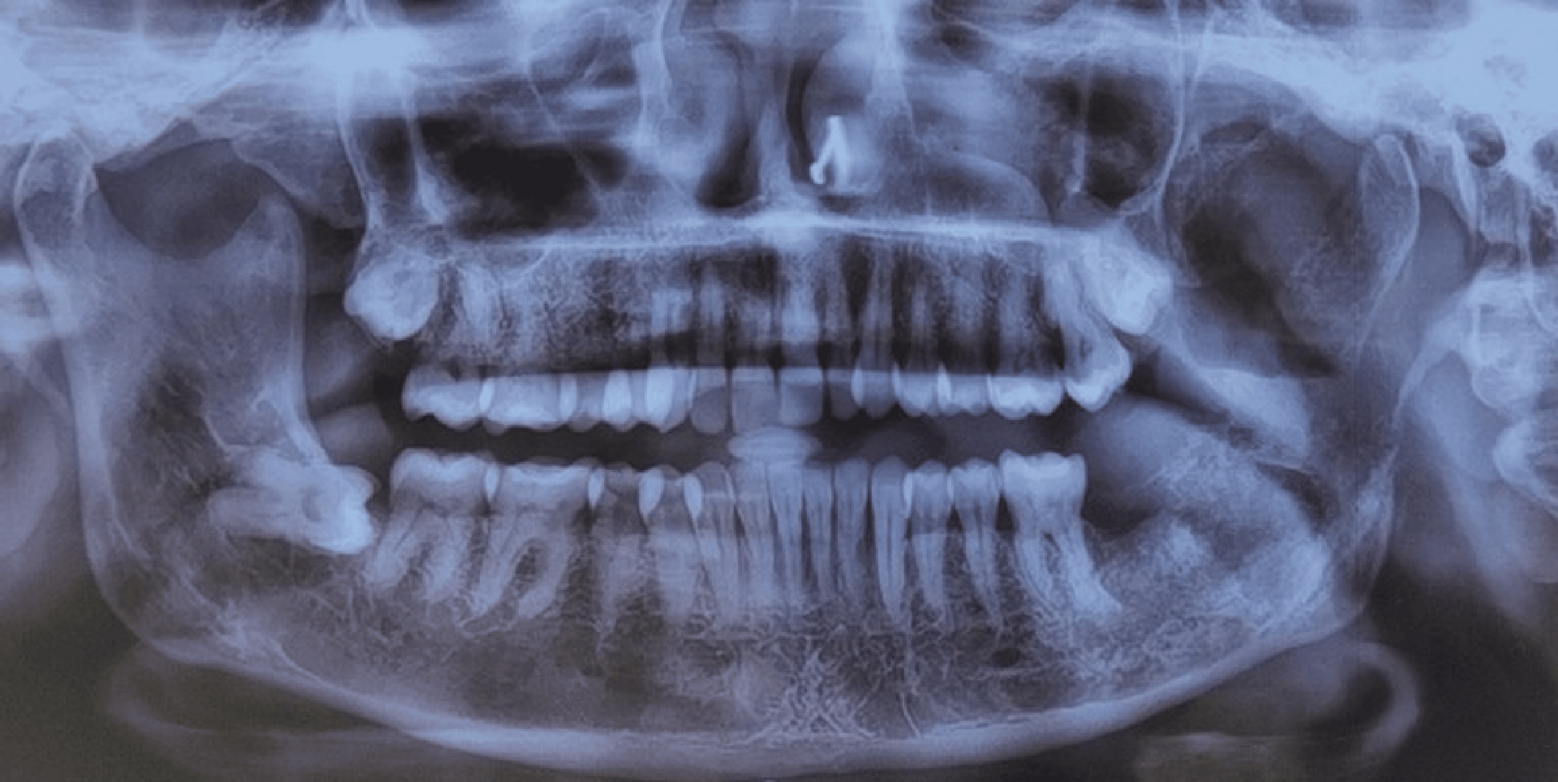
Orthopantomogram four months after the extraction of 37 and 38
